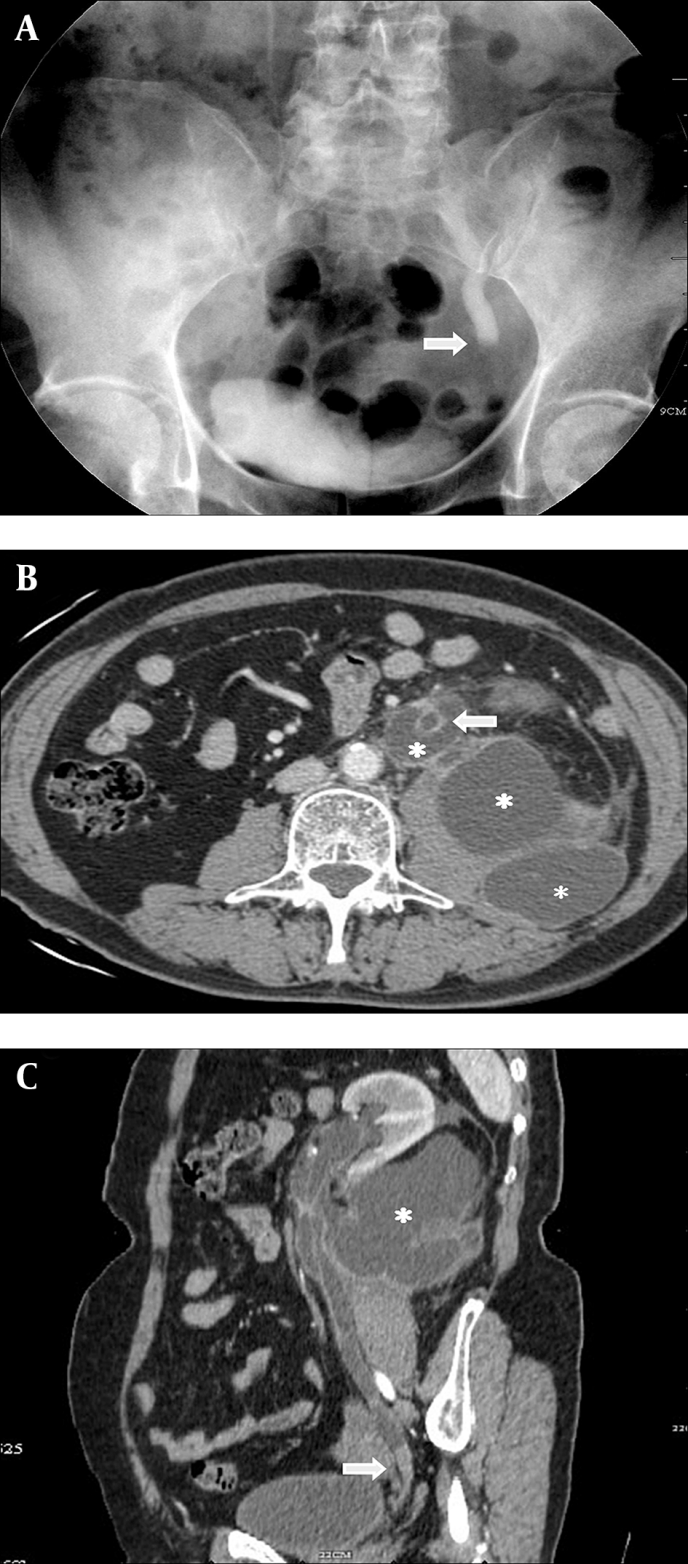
A, The IVU shows the dilated ureter with amputated end (arrow). B, Computed tomography of the abdomen indicates pronounced hydroperinephrosis (asterisk) and dilated ureter (arrow). C, The sagittal oblique reconstruction of left ureter shows a lesion at the end of ureter (arrow) and hydroperinephrosis (asterisk).
